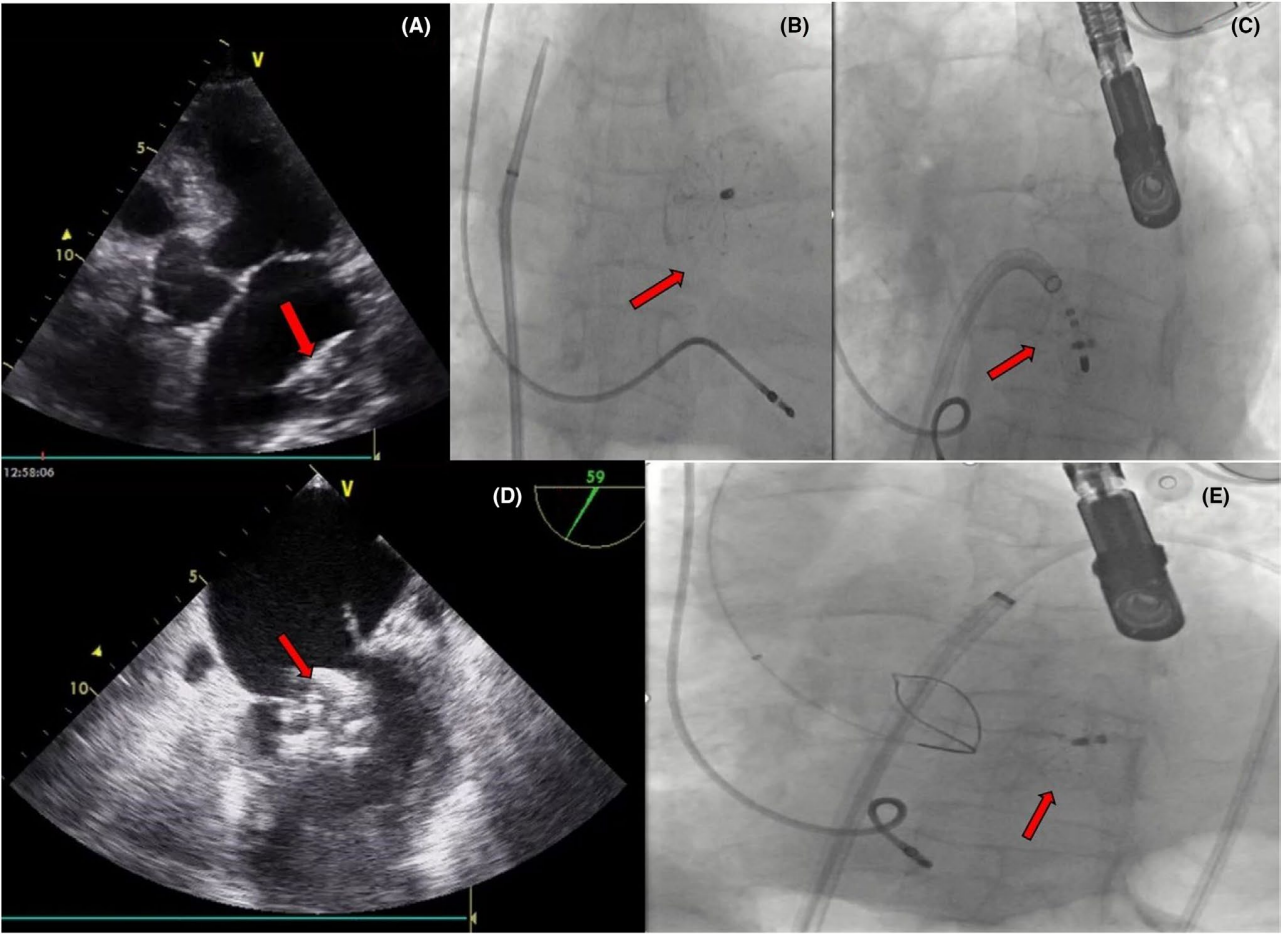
Echocardiographic and fluoroscopic images of the embolized device in Case 2 demonstrate various attempts to retrieve it. (A) T TTE image shows the device embolized in the left atrium. (B) Fluoroscopic image of the device freely floating within the left atrium. (C) An ablation catheter is being used to attempt to push the device into the left ventricle. (D) The device becomes trapped in the mitral valve, leading to acute severe mitral stenosis, as it is being pushed into the left ventricle in preparation for transaortic retrieval. (E) Unsuccessful attempt to capture the device transaortically using a snare catheter.
